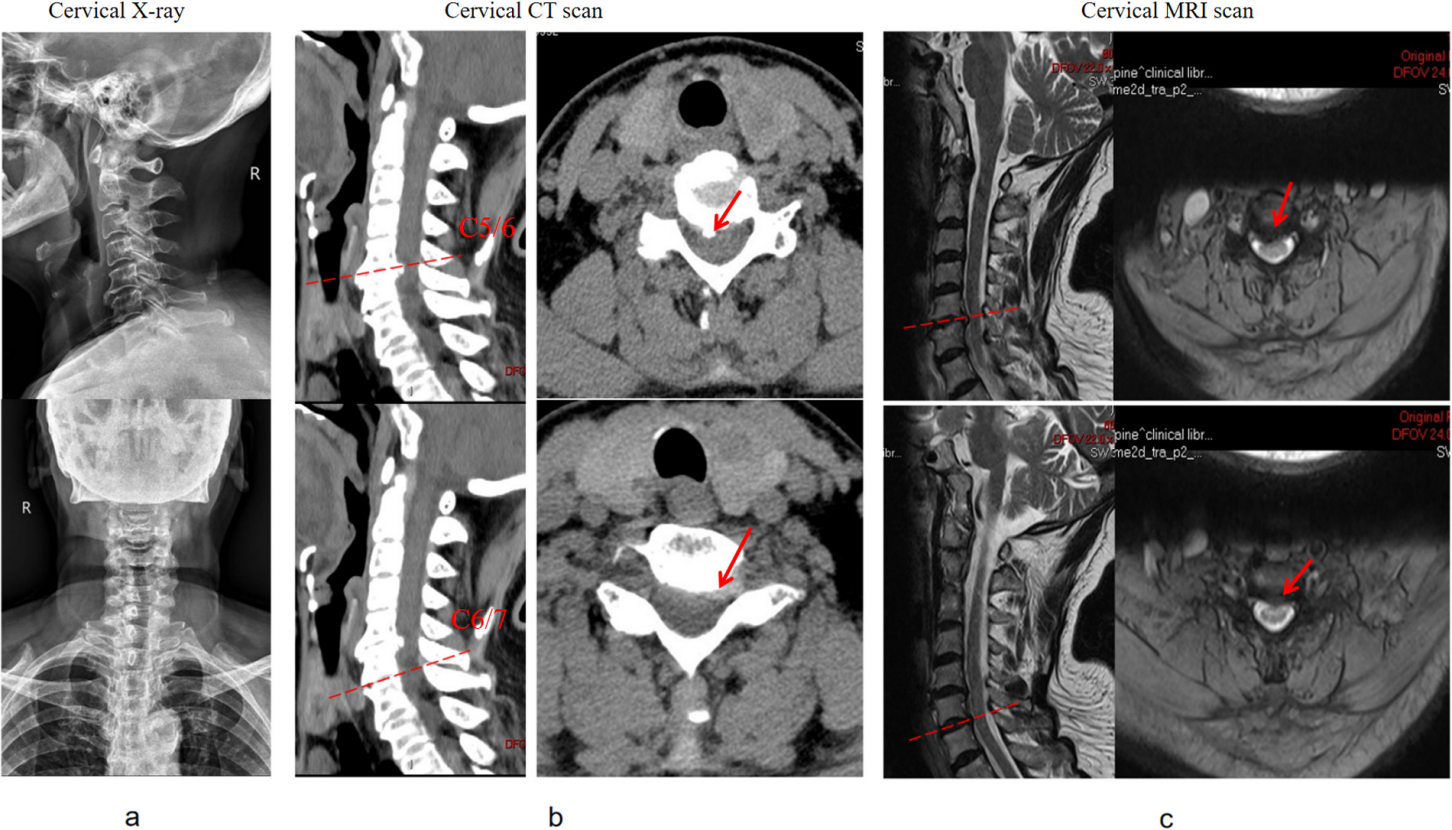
Preoperative imaging examination [including (a) X-ray, (b) CT scan, and (c) MRI] suggested herniation of the C5-7 intervertebral disc with compression of the dural sac and nerve root.
